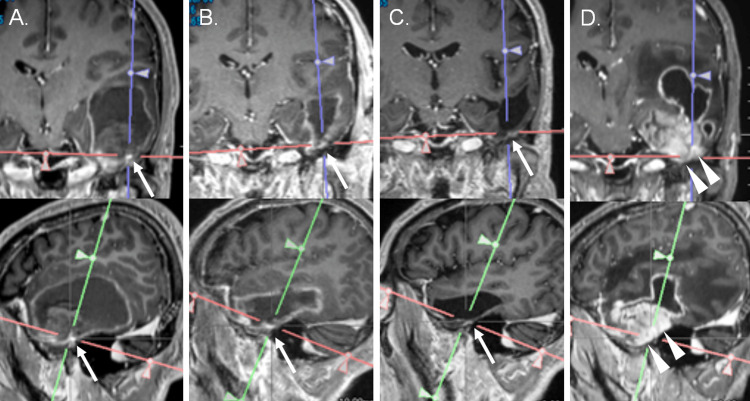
Magnetic resonance (MR) images demonstrating the enhancement around the hematoma and following glioblastoma, reconstructed from three-dimensional gadolinium-enhanced T1-weighted MR images on day five after intracerebral hemorrhage (ICH) (A), immediately after ICH evacuation (B), seven months after ICH (C) and 17 months after ICH (D). There were peripheral linear and nodular (center of the crossbar, arrows in A-C) enhancements on preoperative and postoperative MR images (A and B). The nodular lesion did not change in size and was still enhanced seven months after ICH (C), whereas peripheral linear enhancement disappeared. Irregular mass lesion (arrowheads in D) developed at the corresponding site of the nodular lesion at A–C.
